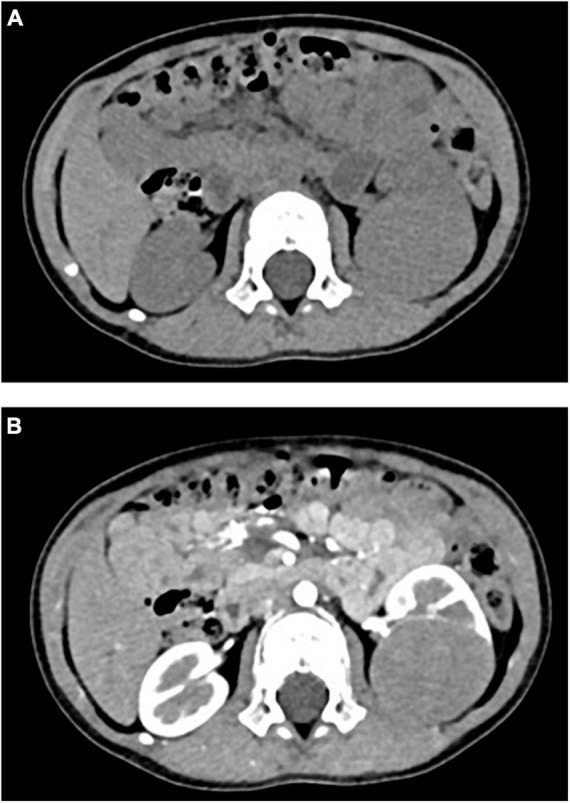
(A) CT plain scan after chemotherapy: CT value was 43HU. (B) CT enhanced after chemotherapy: the third stage showed obvious uneven enhancement, with CT values of about 63HU, 101HU, and 135HU, respectively.
